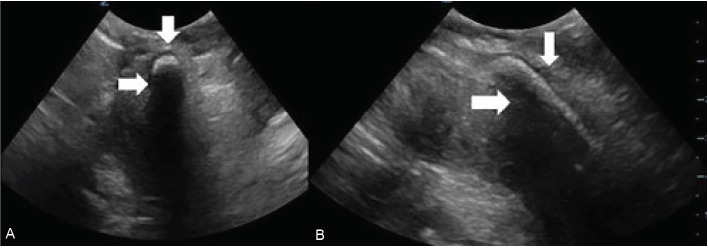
(A) Point-of-care transverse image of the urethra was obtained trans-scrotally. A hyperechoic foreign body (vertical arrow) with shadowing (horizontal arrow) within the penile urethra was identified. (B) Point-of-care ultrasound of the urethra in long axis was obtained trans-scrotally. A hyperechoic foreign body (vertical arrow) with shadowing (horizontal arrow) within the penile urethra was identified.
